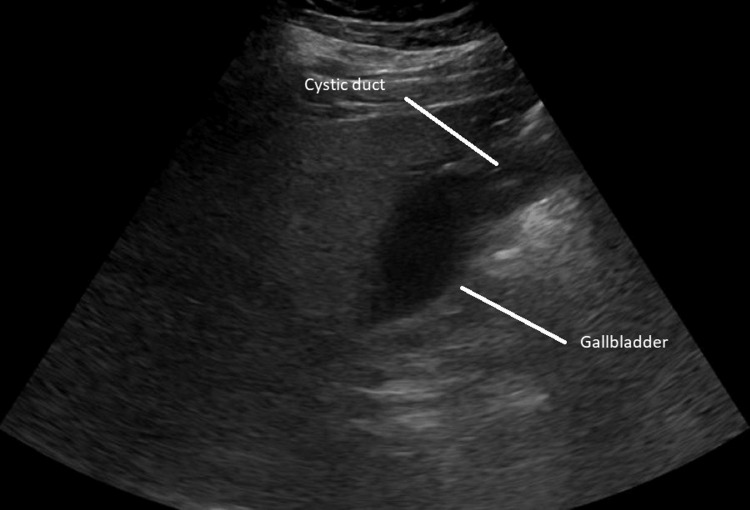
Endoscopic ultrasound completed on admission day two.
